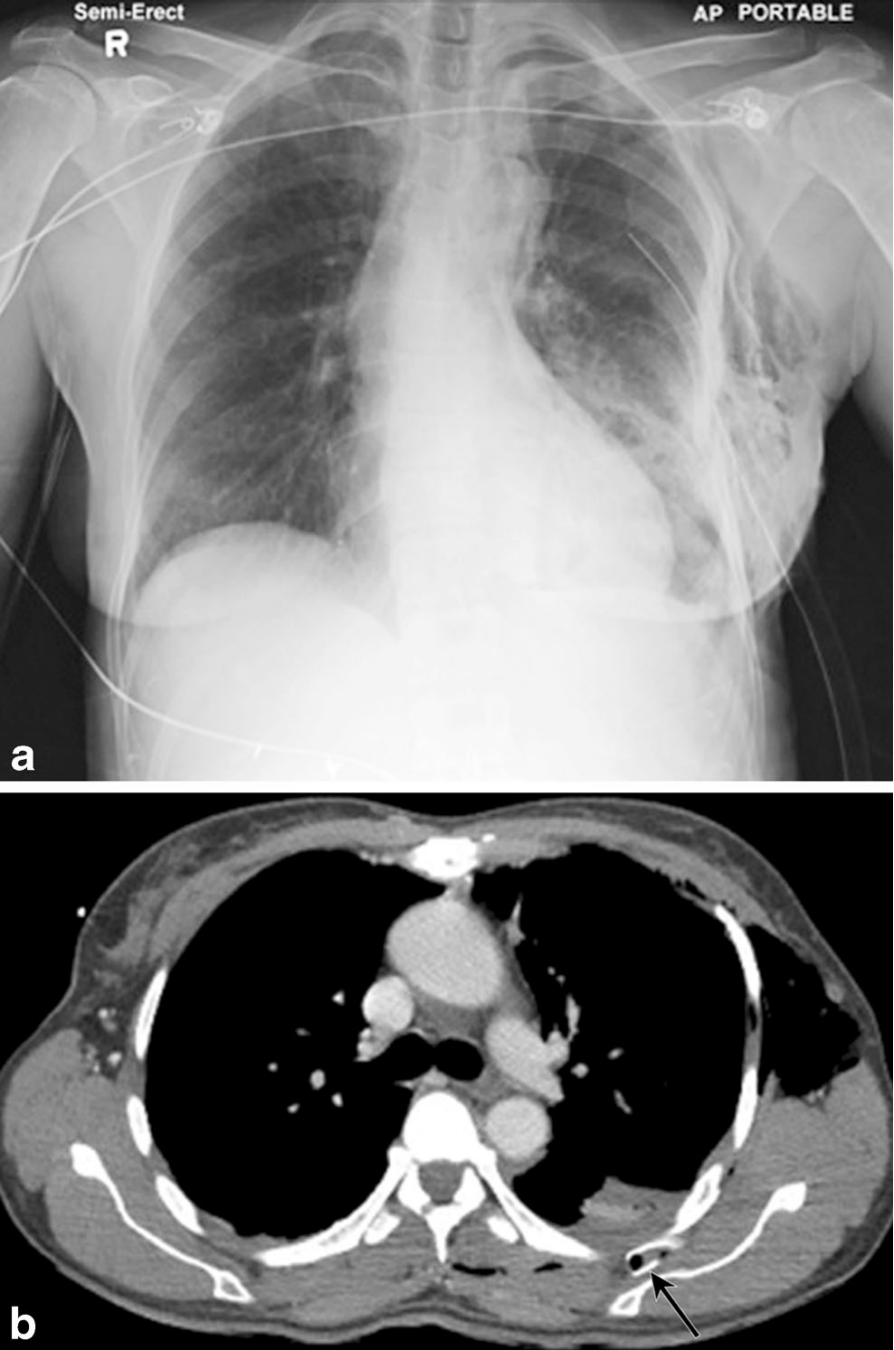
A 52-year-old female status post motor vehicle accident had an emergent chest tube placement by EMR. Semi-erect AP radiograph (a) demonstrates chest tube projecting over the left lateral upper-mid chest; positioning was thought appropriate. However, subsequently performed CT (b) demonstrates chest tube tip within the soft tissues of the posterior chest wall (*black arrow*), between the outer surface of the rib and scapula. Tube was outside the pleural cavity
